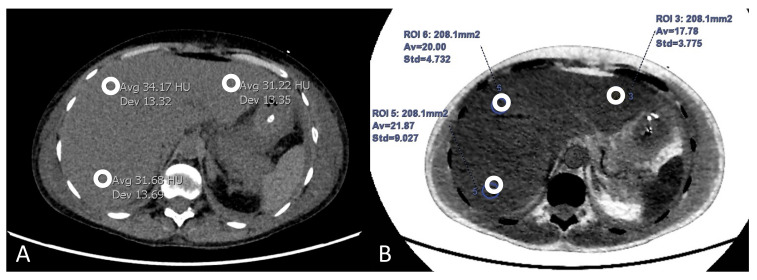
An example of measurements from ROIs placed at three designated liver positions in the left and right liver lobe within the same patient. (A) The HU measurements for a conventional non-contrast CT scan. (B) The corresponding fat percentage measurements made within the AW software on an arterial phase reconstruction. The ROIs in (A,B) are marked with white circles.
